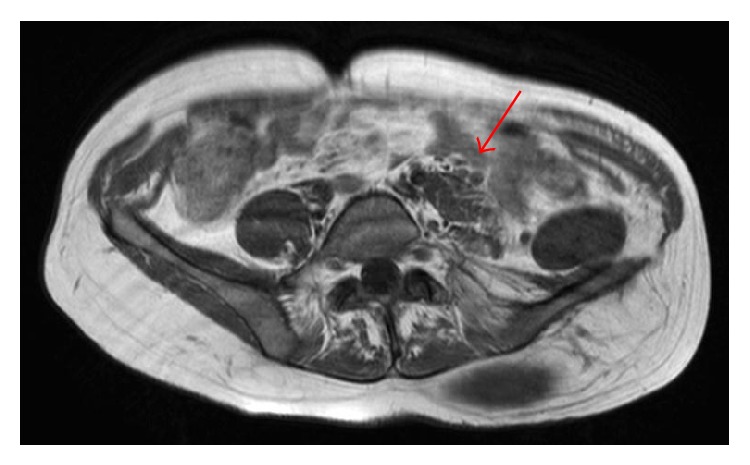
MRI scan showing the retroperitoneal NF1 lesion (arrow) at the bleeding site. Lateral displacement of left psoas muscle due to tumour growth.
